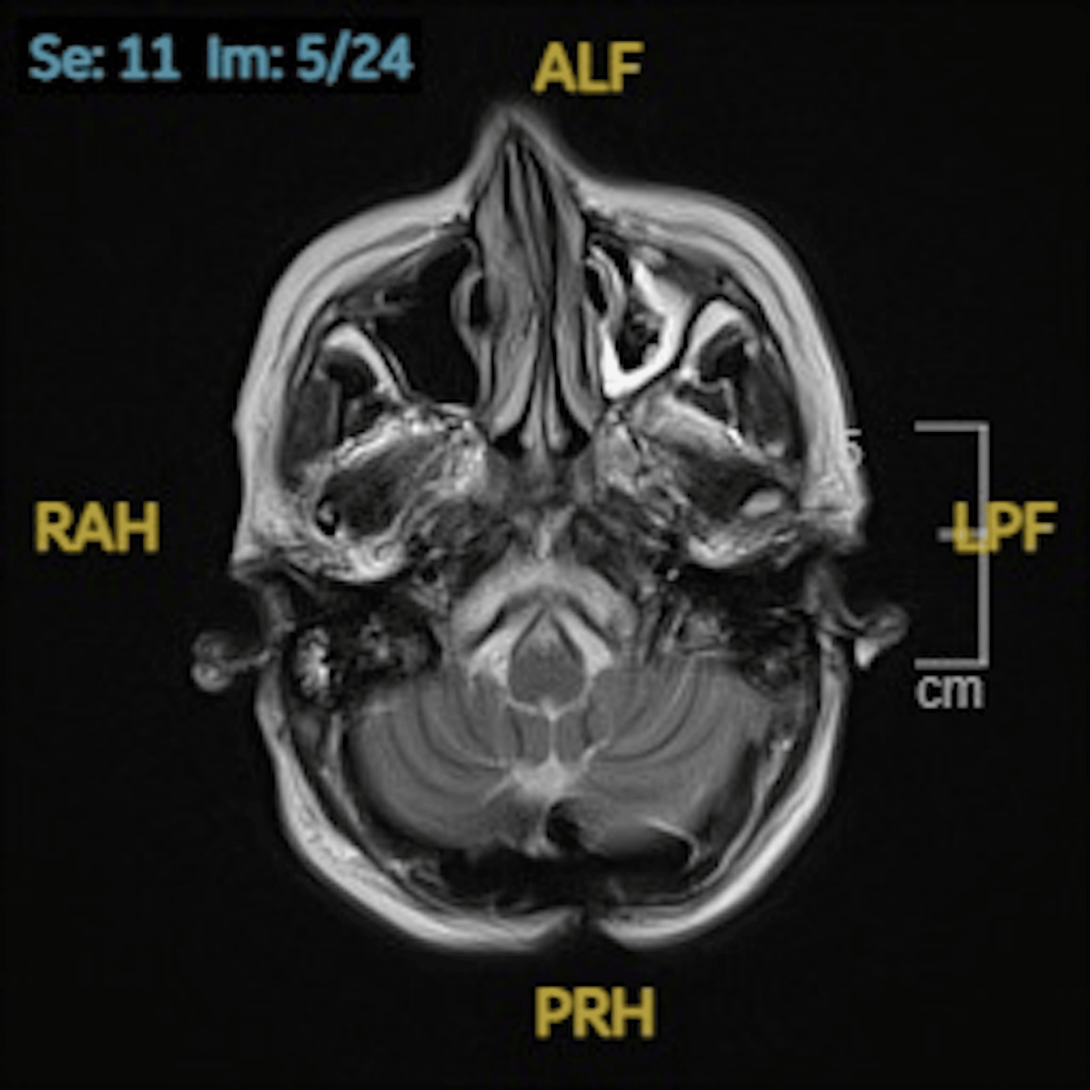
Axial T2-weighted MRI Brain Axial T2-weighted MRI of the brain at the cervicomedullary junction. There is a mild reduction of cerebrospinal fluid in the anterior compartment compared to the posterior compartment. There is no evidence of brain stem compression.
